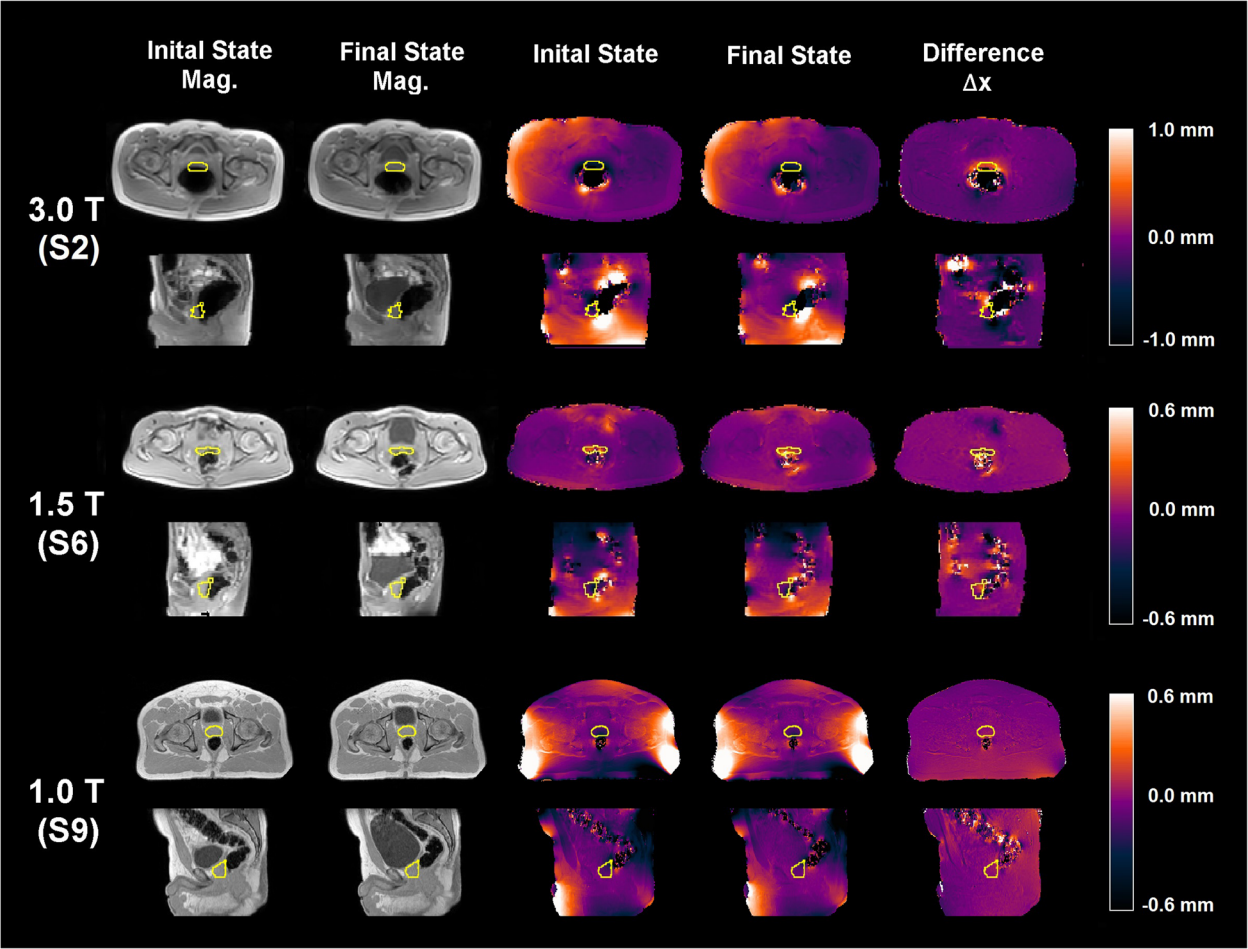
Magnitude images of the dual echo MRI data of initial (column 1) and final (column 2) bladder states for three subjects (S) used to generate subject-induced susceptibility distortion maps. Corresponding B0 maps with off-resonances converted to distortions are shown in columns 3 and 4, respectively. Clinical target volumes (CTVs, prostate + proximal 1 cm of seminal vesicles) are delineated. Distortion difference maps (difference ∆X) are shown in the final column (final minus initial) with the distortion scale shown in millimeters
